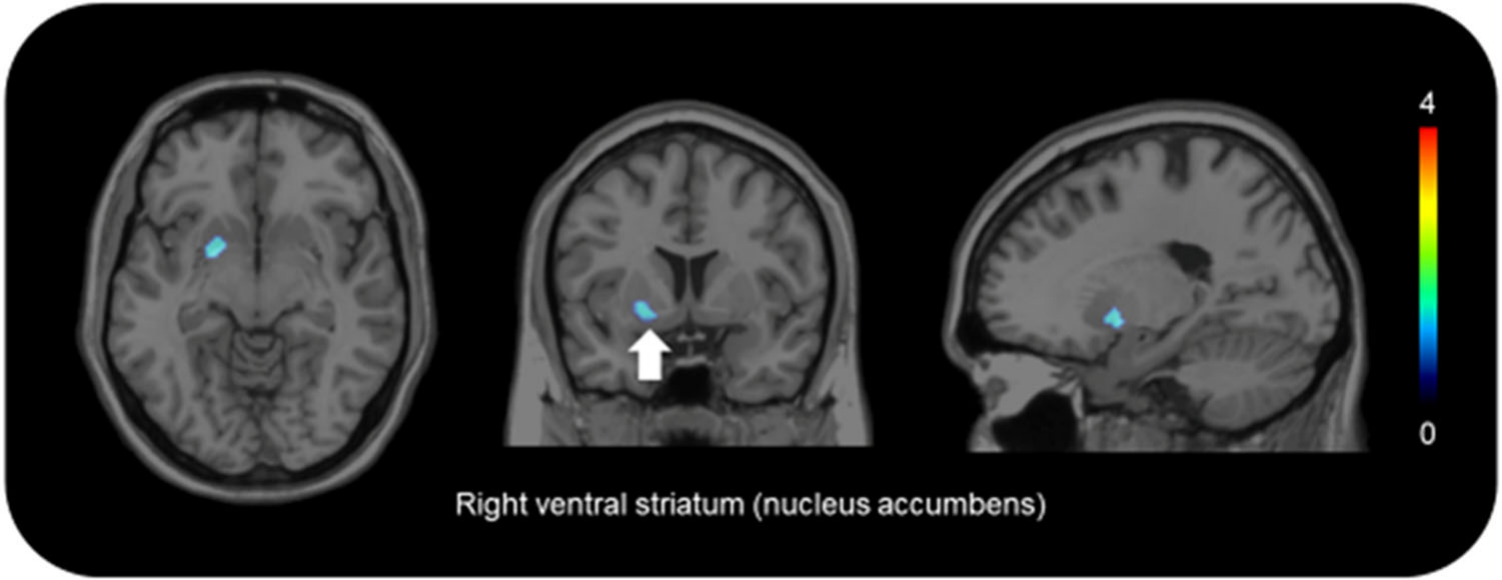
Brain region of increased dopamine release The brain region superimposed on magnetic resonance images shows marked reduction in [^11^C]-raclopride binding after active stimulation. The color bar shows the *t* value
